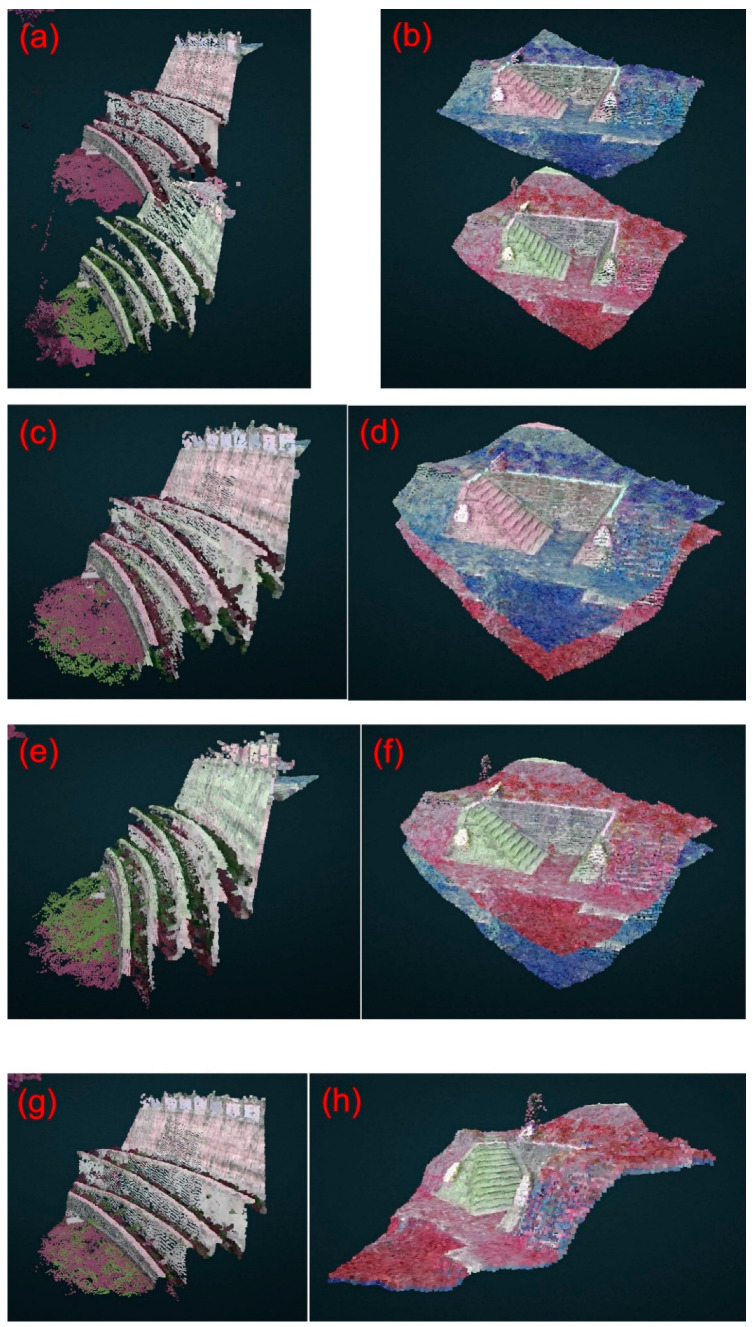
The partial integration results of point cloud Models 3-1 and 3-2 in study area 3: (a,b) without the CT method, (c,d) with the 4-parameter CT method, (e,f) with the 6-parameter CT method, and (g,h) with the 7-parameter CT method. The entire point cloud fusion results for (a–d) can be found at the following four links (accessed on 30 August 2024): https://www.pointbox.xyz/clouds/66b37adb817a4275f75567fc, https://www.pointbox.xyz/clouds/66b37cd3817a42f55d5567fe, https://www.pointbox.xyz/clouds/66b37dcc817a428987556800, https://www.pointbox.xyz/clouds/66b37de0817a423873556802.
